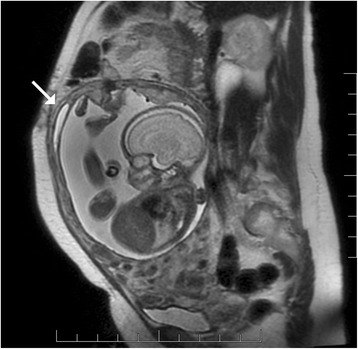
MRI showing 4 mm fundal uterine wall thickness and 0.7 cm by 5 cm amniotic fluid leak (arrow)
